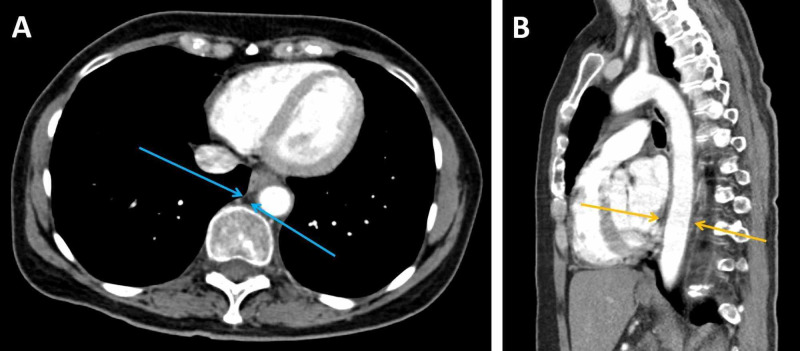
Follow-up CT imaging of the thorax, abdomen and pelvis performed six weeks after discharge (A: transverse plane, arterial phase; B: sagittal plane, arterial phase) A: This image shows resolution of the peri-aortic haematoma and restoration of the normal mediastinal fat in the posterior mediastinum (blue arrows) B: This image shows a normal calibre aorta after resolution of the compressive haematoma (yellow arrows)
